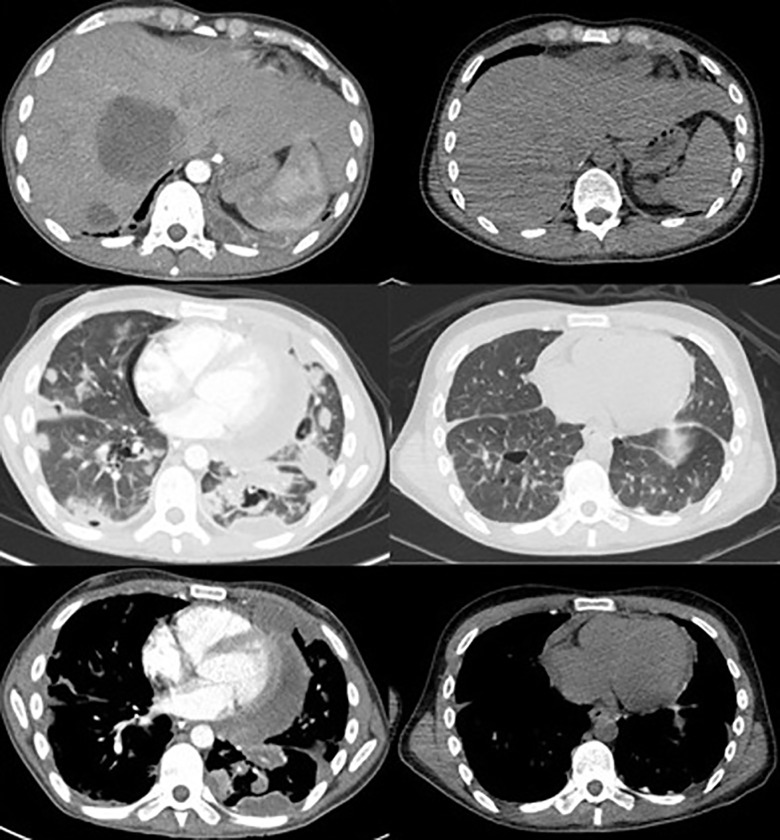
Figure showing response of disease after four cycles of pembrolizumab. Pretreatment CT image (*left*) and PET-CT scan after four cycles of pembrolizumab (*right*). The *top* row shows a reduction in the size of liver metastases; the *middle* row shows a reduction in the size of lung metastatic deposits; and the *bottom* row shows the mediastinal response.
